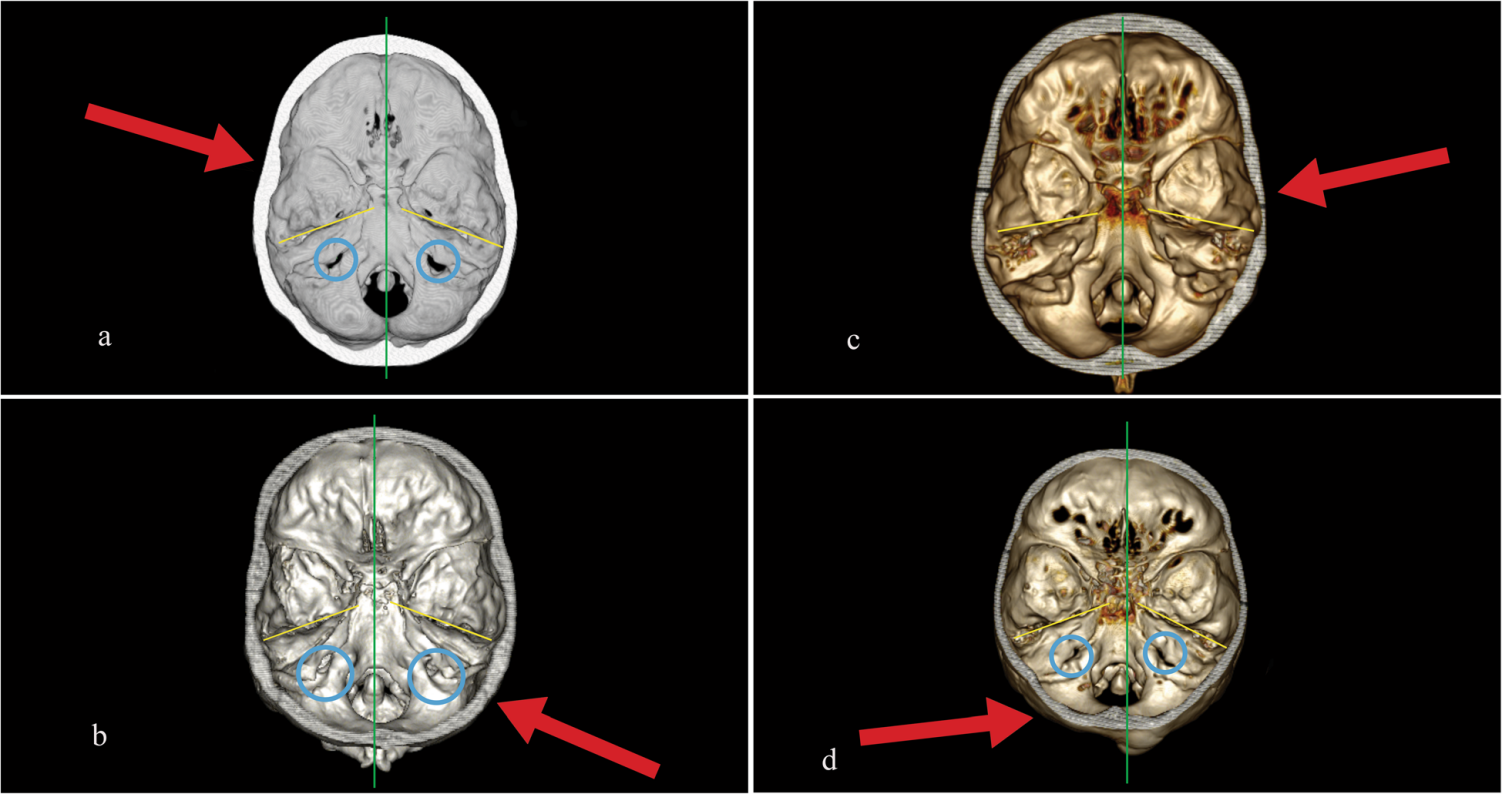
a Three-dimensional computed tomography (3D CT) of a 23-year-old woman 1 year after head trauma on the left temporal bone. Symptoms: tension-type headache on the left side, dizziness, chronic undernourishment. b 3D CT of a 43-year-old man 10 years after head trauma on the right temporo-occipital suture. Symptoms: severe tension-type headache on the right side, cervical pain. c 3D CT of a 41-year-old woman 1 month after head trauma on the temporal bone. Symptoms: dizziness. d 3D CT of a 47-year-old woman 3 years after trauma on the occipital bone. Symptoms: severe tension-type headache, dizziness, tinnitus. The red arrow shows the direction and the localisation of head impact. The green vertical axis allows us to see the asymmetry of the skull base. The blue rings show the size of the jugular foramen. The yellow lines appreciate the differences in the position of the petrous temporal bone
